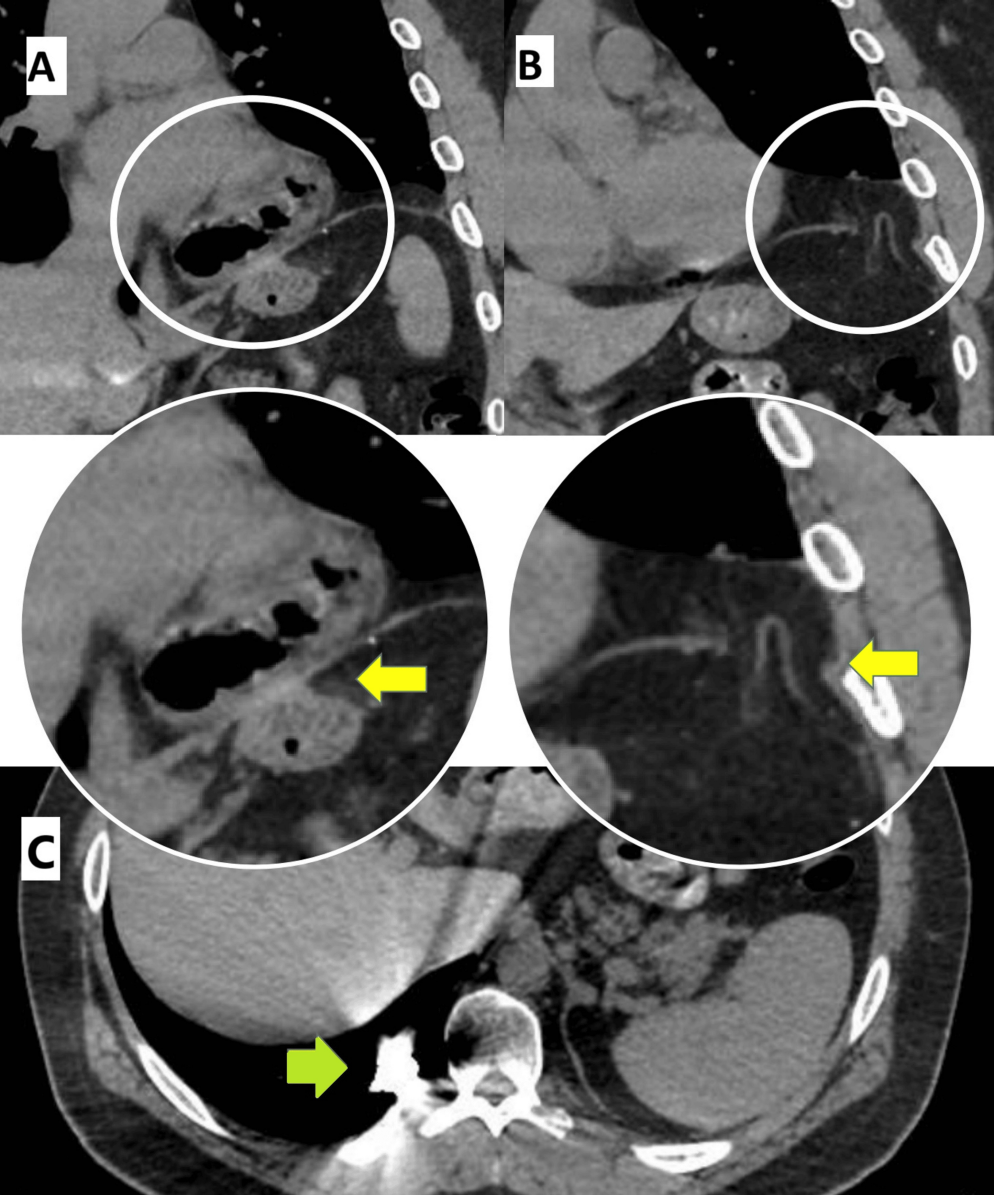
Thoracic and abdominal CT scan A. Voluminous left parahiatal diaphragmatic hernia with gastric fundus in the thoracic cavity (yellow arrow). B. Left subcostal diaphragmatic hernia with adipose content (yellow arrow). C. Metal density image in the posterobasal segment of the right lower lobe (green arrow).
